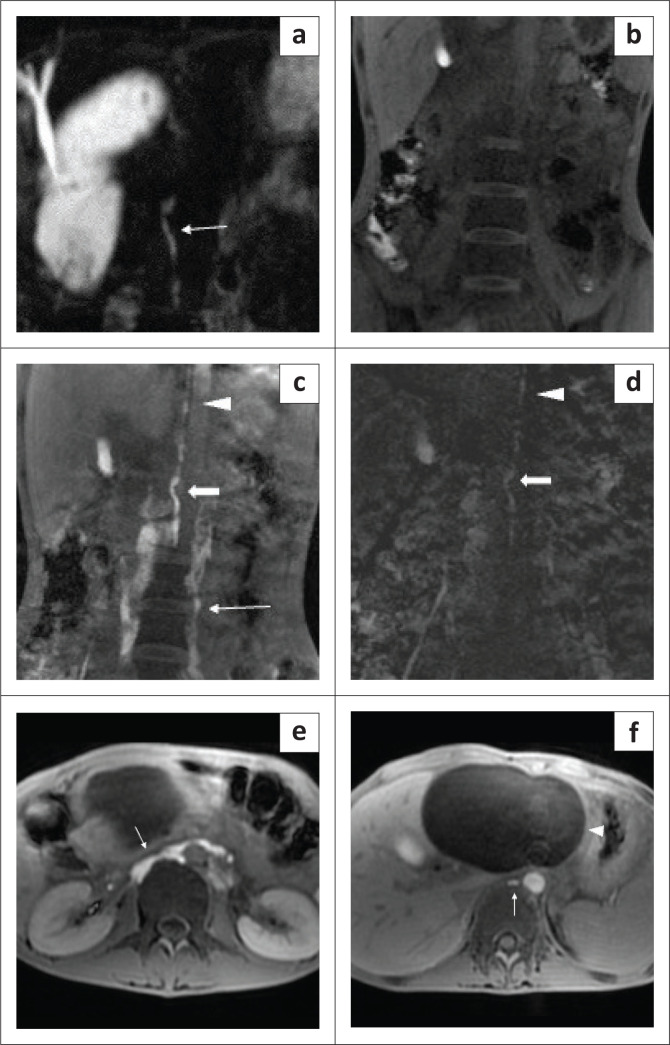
MR lymphangiography in a 15-year-old child with postoperative chylous ascites. The central conducting lymphatics were interpreted as normal in this child. (a) Coronal heavily T2-weighted MRI images of the abdomen with thin Maximum Intensity Projection (MIP) reconstruction depicted a normal cisterna chyli (white arrow). (b) Coronal pre-contrast T1-weighted image of abdomen and pelvis and (c) coronal T1-weighted image of abdomen and pelvis at 7 min after nodal contrast injection demonstrated normal opacification of the cisterna chyli (bold arrow) with faint opacification of the thoracic duct (arrow head). Contrast was still visible in the lumbar lymphatics (arrow). No intraperitoneal leak of contrast was seen. (d) The central conducting lymphatics were highlighted in the subtracted image depicting the cisterna chyli (bold arrow) and thoracic duct (arrow head). (e) Axial T1-weighted image 5 min after intranodal contrast administration showed confluence of the bilateral lumbar lymphatics to form the cisterna chyli (arrow) at the level of renal hilum. (f) Axial T1-weighted image of the upper abdomen 9 min after intranodal contrast administration depicted a normal thoracic duct (arrow). A pancreatic pseudocyst (arrow head) was seen anterior to it.
